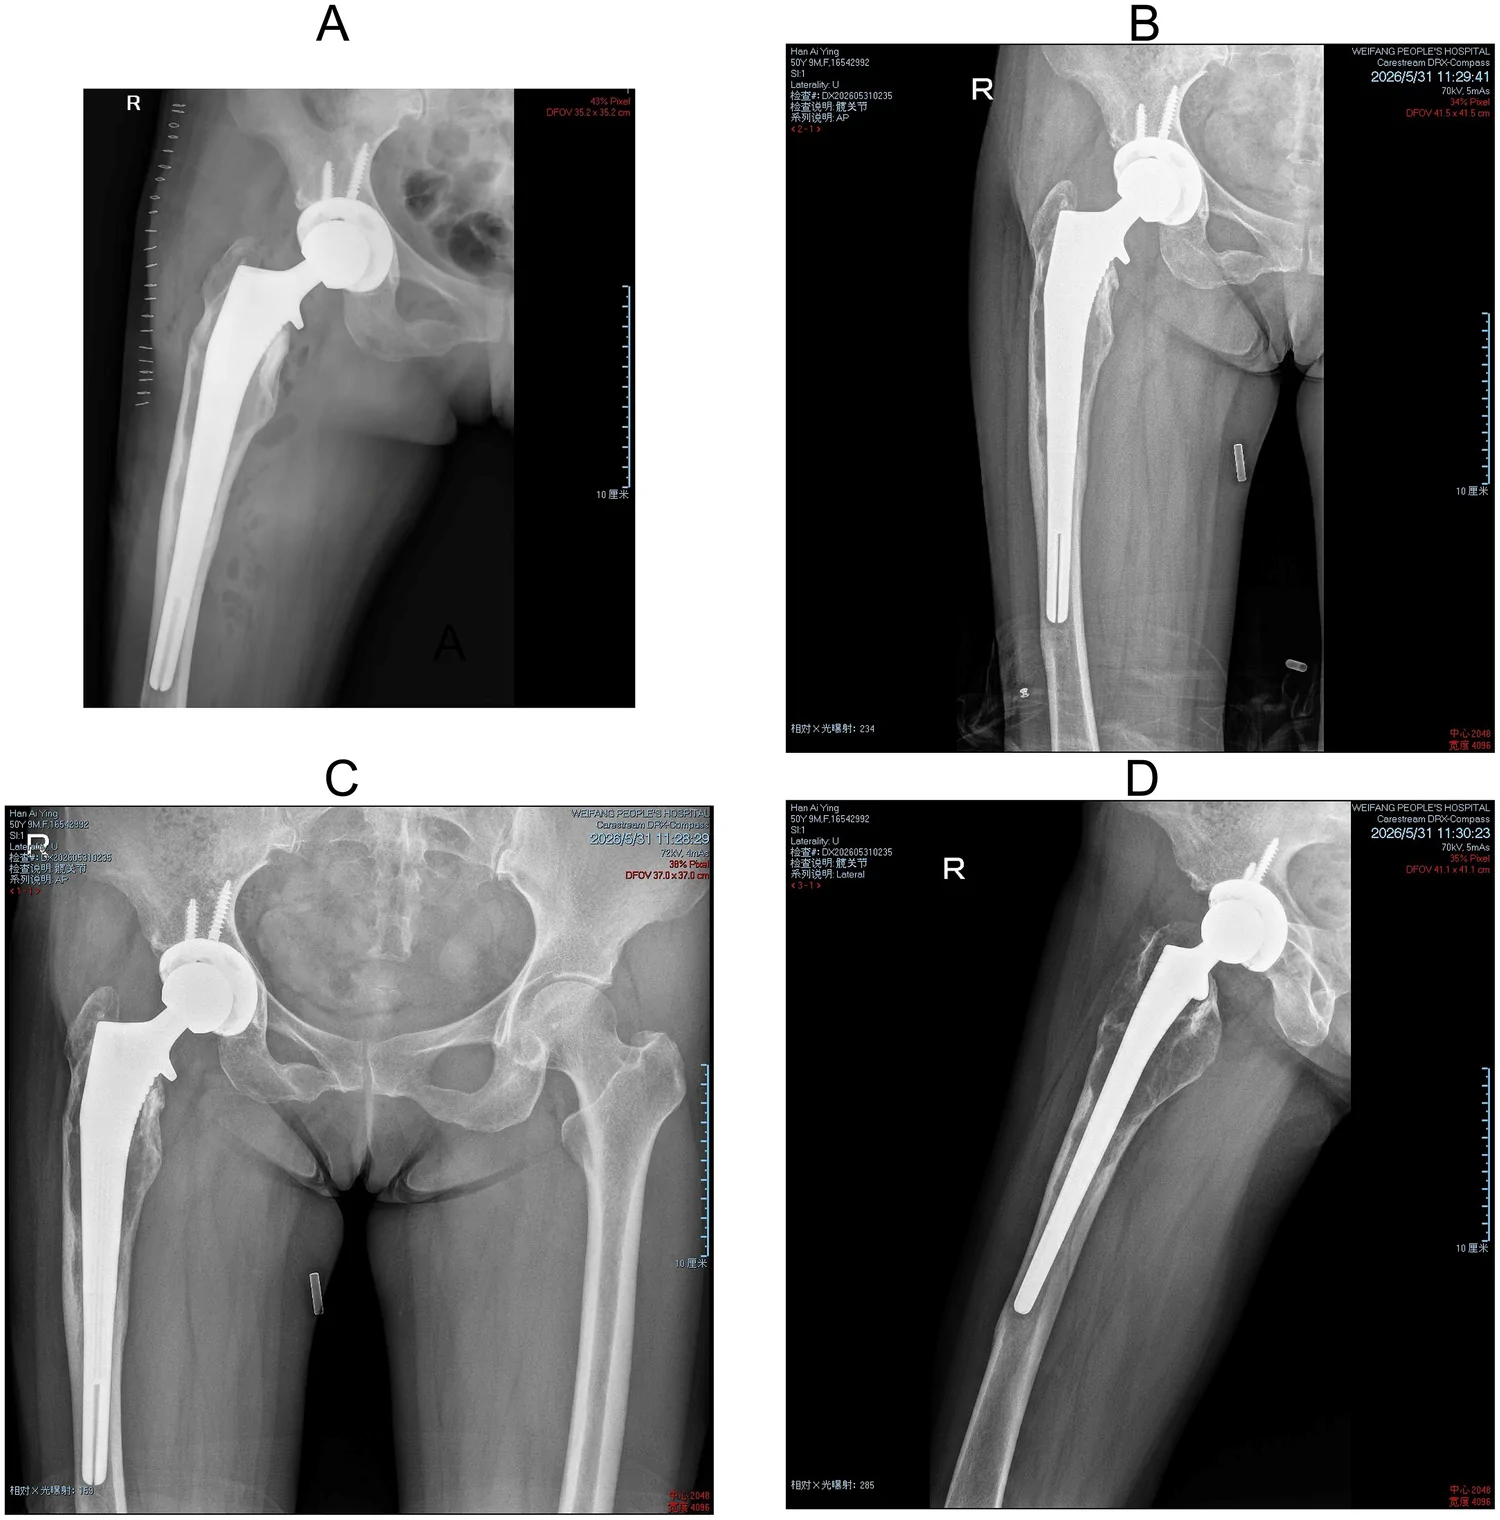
Postoperative and follow-up radiographs. (A) Anteroposterior radiograph of the right hip obtained immediately after surgery, showing appropriate alignment and fixation of the cementless revision stem and acetabular component. (B–D) Anteroposterior and lateral radiographs at 6-month follow-up, demonstrating stable implant fixation without evidence of subsidence, periprosthetic radiolucency, or loosening.
